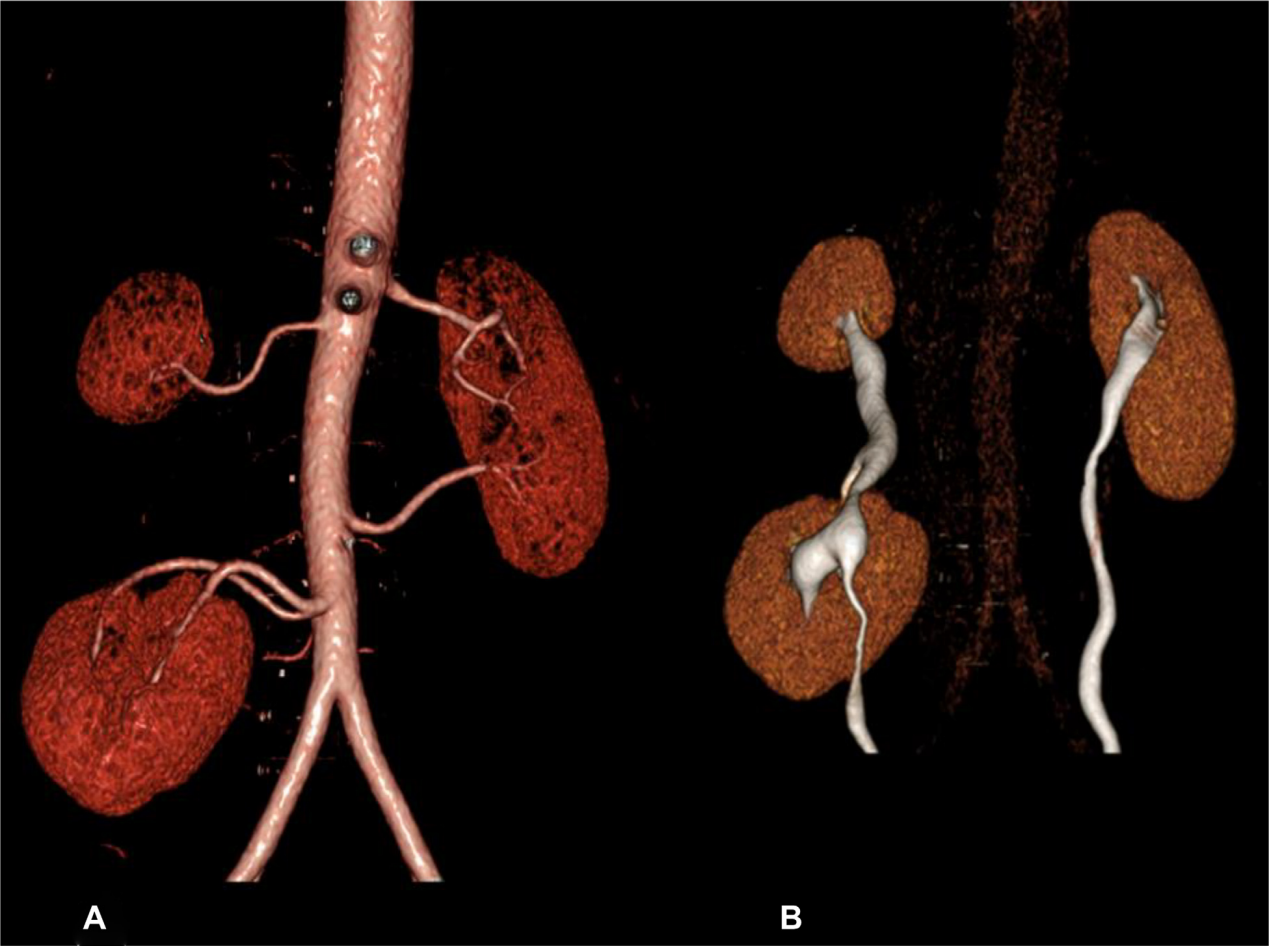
(A) Volume rendering image of CT abdomen and pelvis study demonstrates the arterial supply for the 3 kidneys. The native right kidney, which is caudally placed, is supplied by early bifurcated renal artery arising at lower margin of L3 vertebral body. The supernumerary kidney, which is cranially placed, is separately supplied by a renal artery arising at the level of L1 vertebra. The left kidney is supplied by 2 renal arteries (B) Volume rendering image shows the 3 kidneys are excreting symmetrically with the right superiorly located smaller kidney's (supernumerary) ureter joining the right collecting system at the pelvic-ureteric junction. A single ureter is then draining into the urinary bladder. Note the right native kidney has malrotated pelvis facing anteriorly.
